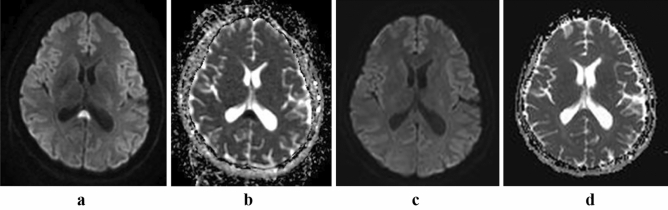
Type B acute MBD. Axial MRI scans displayed a focal lesion in the splenium, showing symmetrical hyperintensity on DWI (a) and hypointensity on the ADC map (b). Seven days after treatment, the lesion disappeared on the DWI (c) and ADC map (d).
